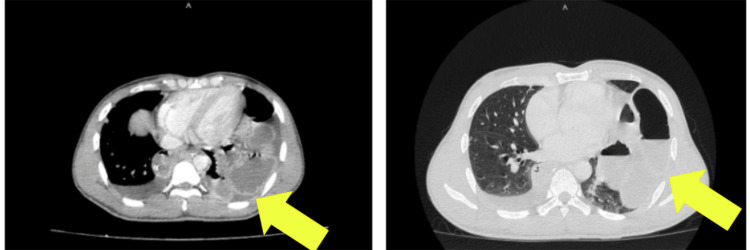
Computed Tomography (CT scan) Thorax, Abdomen and Pelvis - intravenous contrast (right picture), lung window (left picture) Noted the absence of left hemidiaphragm associated with herniation of the bowel (likely dilated jejunum of 5.2 cm in diameter), mesenteric vessels and fat into the left thoracic cavity from the abdominal cavity. There was also mesentery fluid and interloop free fluid adjacent to the herniated bowel. These imaging findings confirmed the left diaphragmatic hernia with signs suggestive of strangulation.
